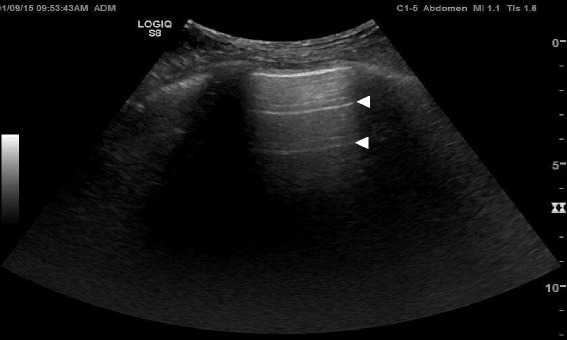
A-lines. A-lines (*arrowheads*) are horizontal artifacts generated by the repeated reflection of the ultrasound beam between the pleural line and the probe surface
